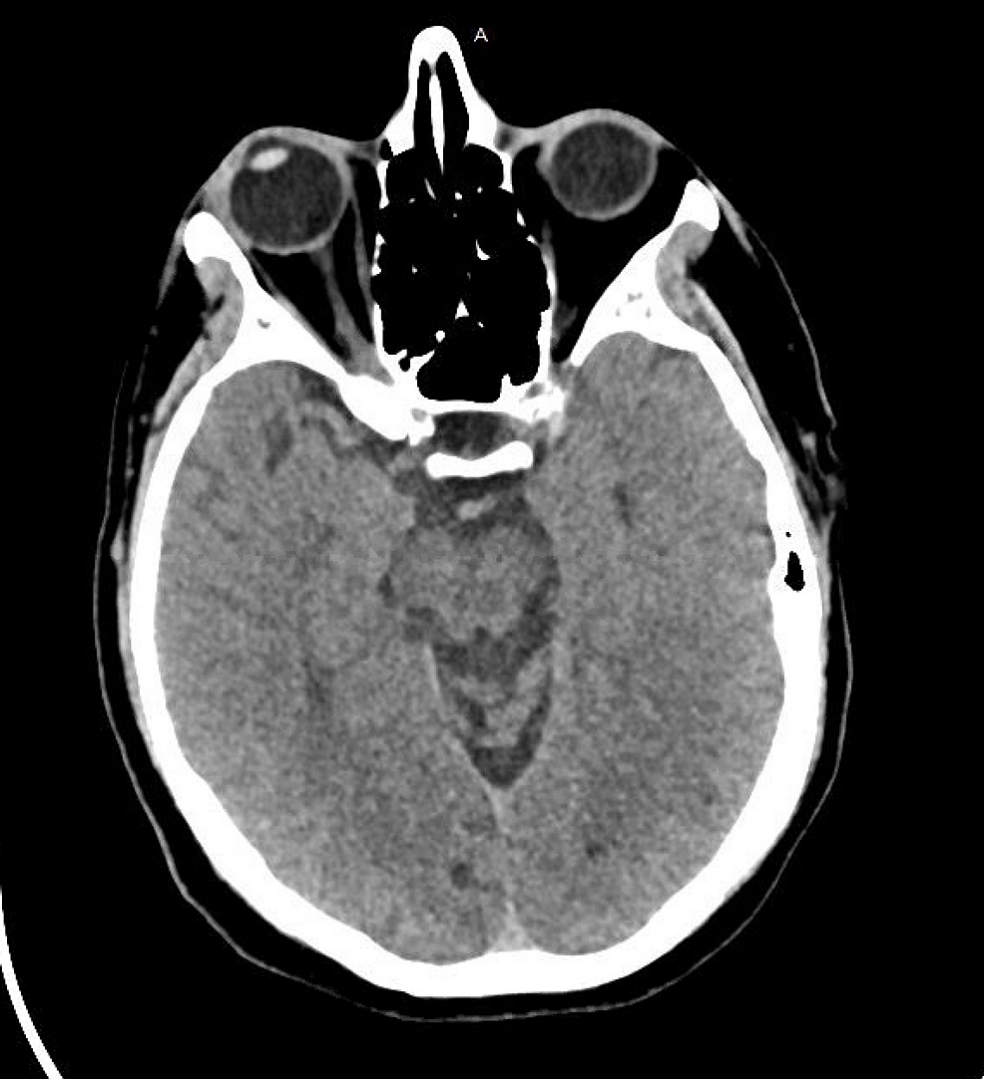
Initial CT of the head without intravenous contrast: no intracranial mass, mass effect, midline shift, or herniation is present. No acute intracranial hemorrhage is present. No findings of an acute territorial infarction are present. No extra-axial collection is present. The ventricular system is normal in size. Brain parenchymal attenuation is normal.
